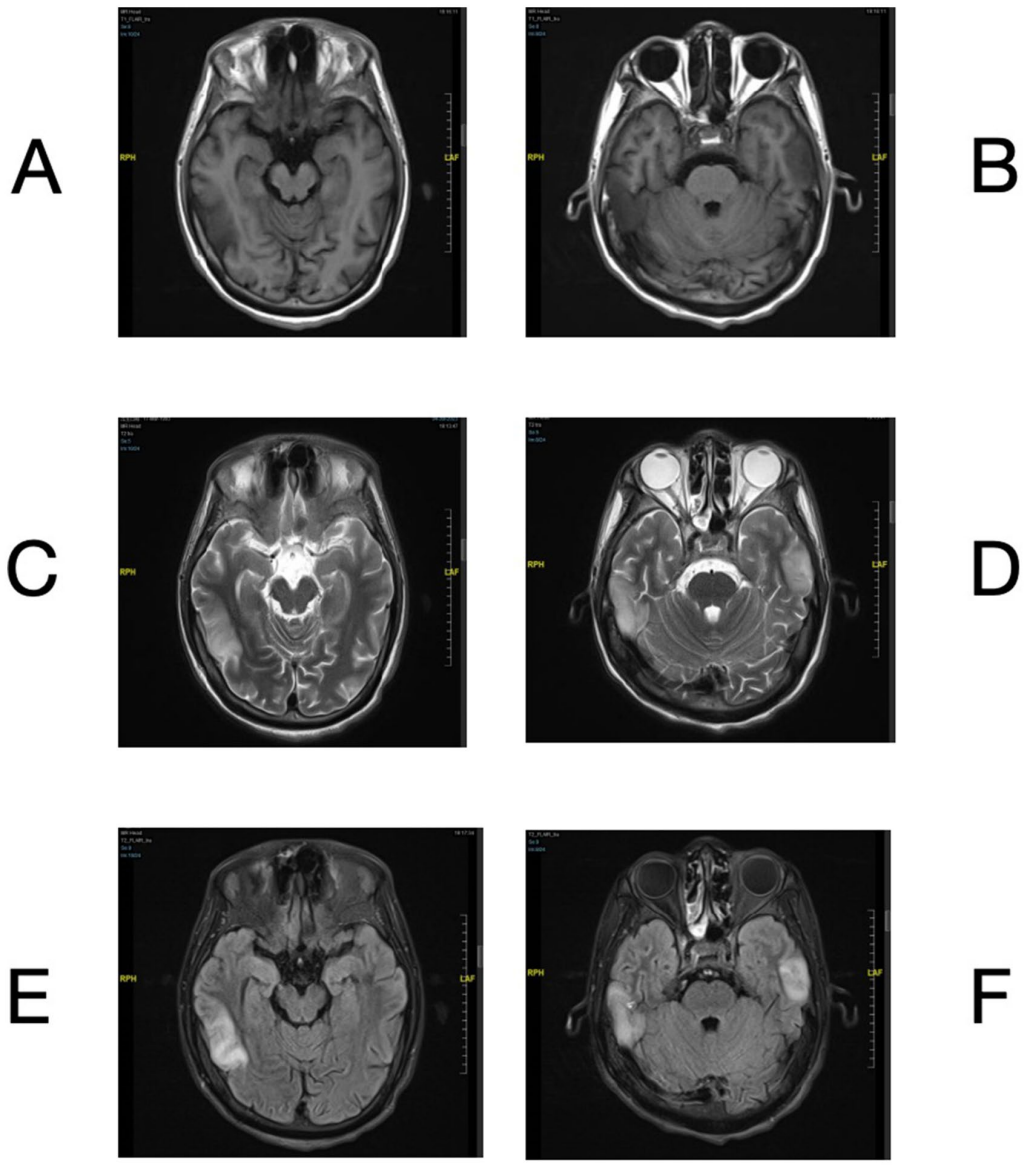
Brain MRI of bilateral temporal lobe lesions. Brain MRI showed T1 hypointensity (A,B) and T2 hyperintensity (C,D) in bilateral temporal lobes. The lesions exhibited hyperintensity on the FLAIR sequence (E,F).
